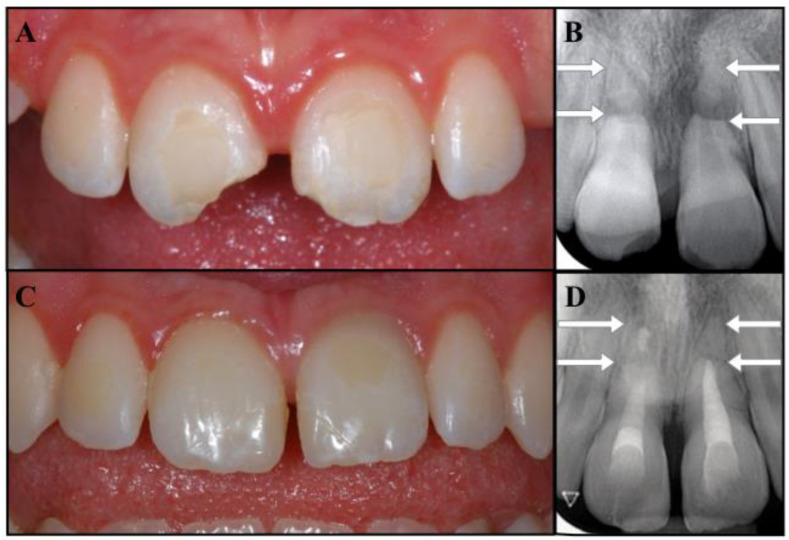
(A,B) Clinical photograph and intraoral radiographs of teeth #1.1 and #2.1 at the initial stage of treatment (replantation) and at the 30-month follow-up. (C,D) The images show good coronal restorations, mild signs of discoloration in the cervical portion of tooth #2.1, healing in the fracture lines, a healthy periapex.
